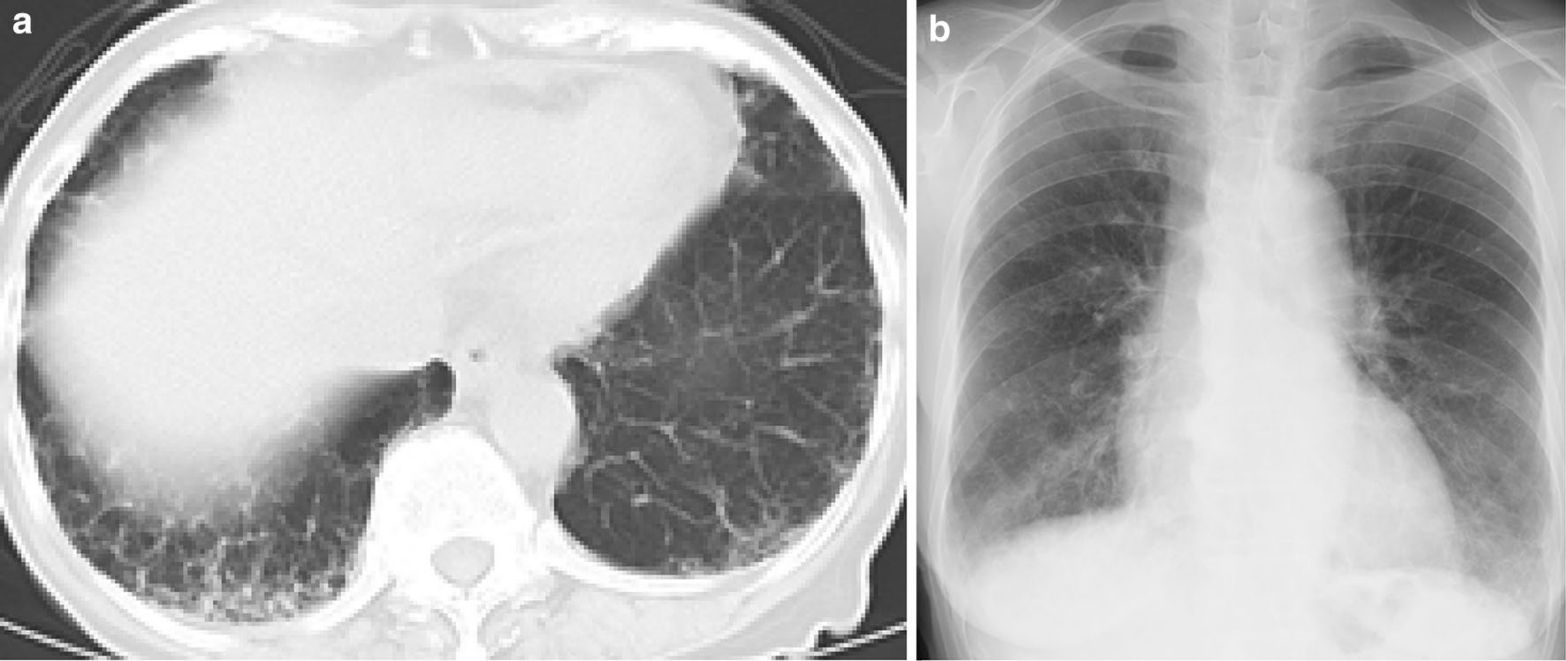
Chest CT and chest X-ray before treatment with biologics. a Chest CT was performed 10 month before admission. Mild reticular shadow was observed in the bilateral lower lung. b Chest X-ray was performed 2 month before admission. Mild reticular shadow was observed in the bilateral lower lung again
